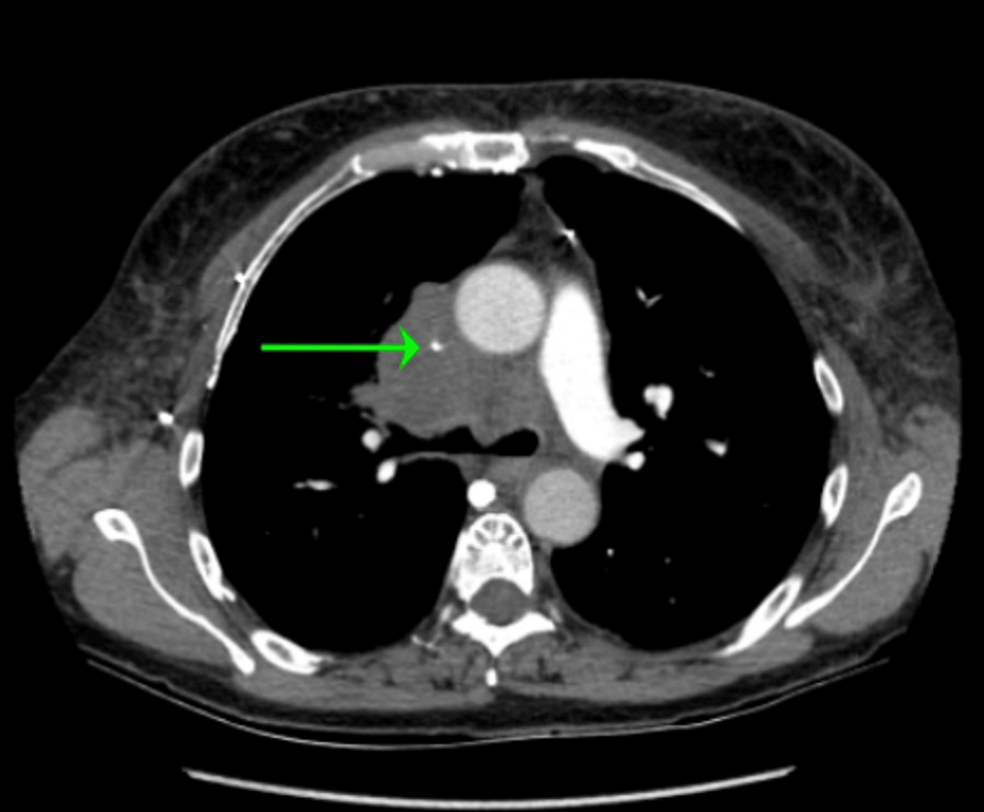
CTPA (axial view) showing severe narrowing of the SVC from compression by the right suprahilar mass CTPA: computed tomography pulmonary angiography; SVC: superior vena cava
